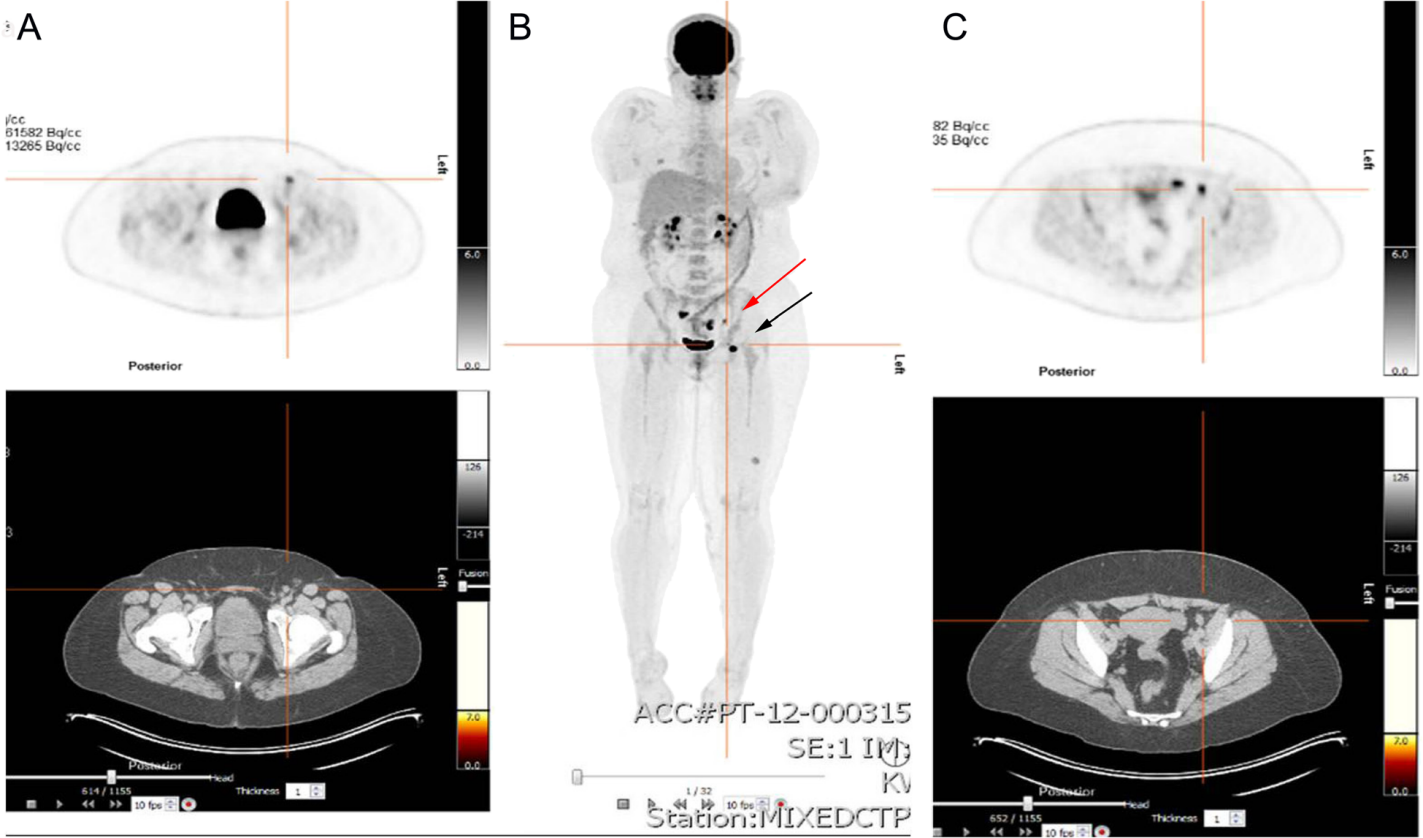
FDG PET and CT images (A) Axial FDG PET and CT images demonstrating intense avidity in a left superficial inguinal lymph node; (B) Whole body FDG PET demonstrating intense avidity in three inguinal superficial lymph nodes (black arrow) and left deep inguinal (external iliac) lymph node (red arrow). Hypermetabolism is seen in the left thigh biopsy site and likely physiologic changes in the right and left adnexae. (C) Axial FDG PET and CT images demonstrating the avid left deep inguinal lymph node.
